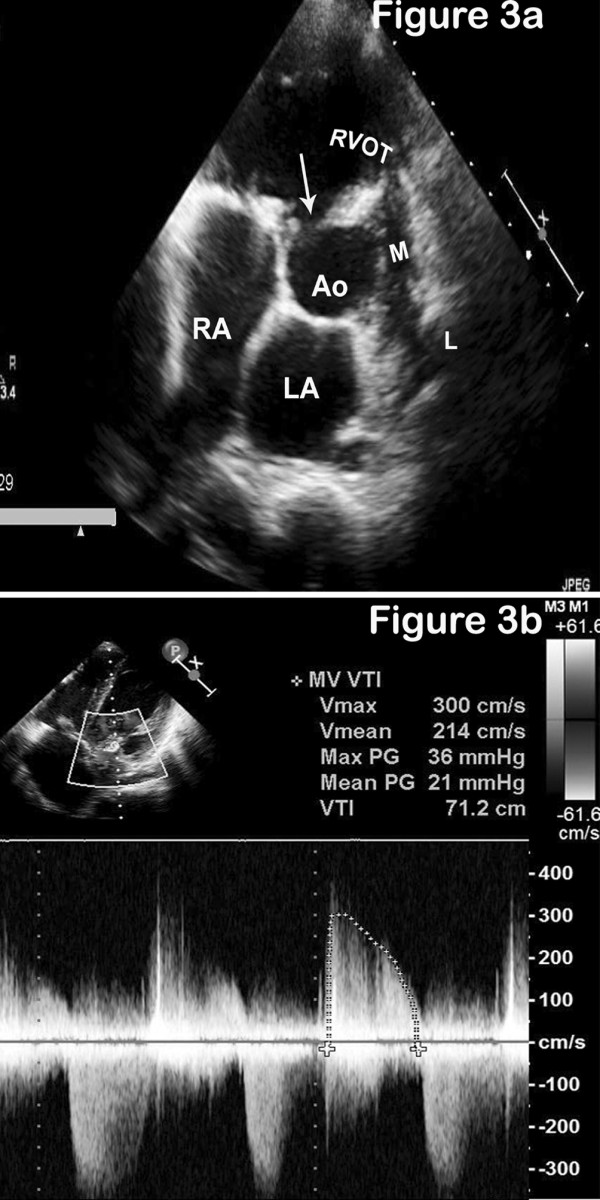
RVOT morphology and Doppler study of the mitral valve. (A) Short axis image demonstrating the subaortic ventricular septal defect (arrow), hypoplastic right ventricular outflow tract (RVOT), main pulmonary artery (M) with confluent branch pulmonary arteries. L, left pulmonary artery; RA, right atrium; LA, left atrium. (B) Image demonstrating Doppler gradients across the mitral valve.
